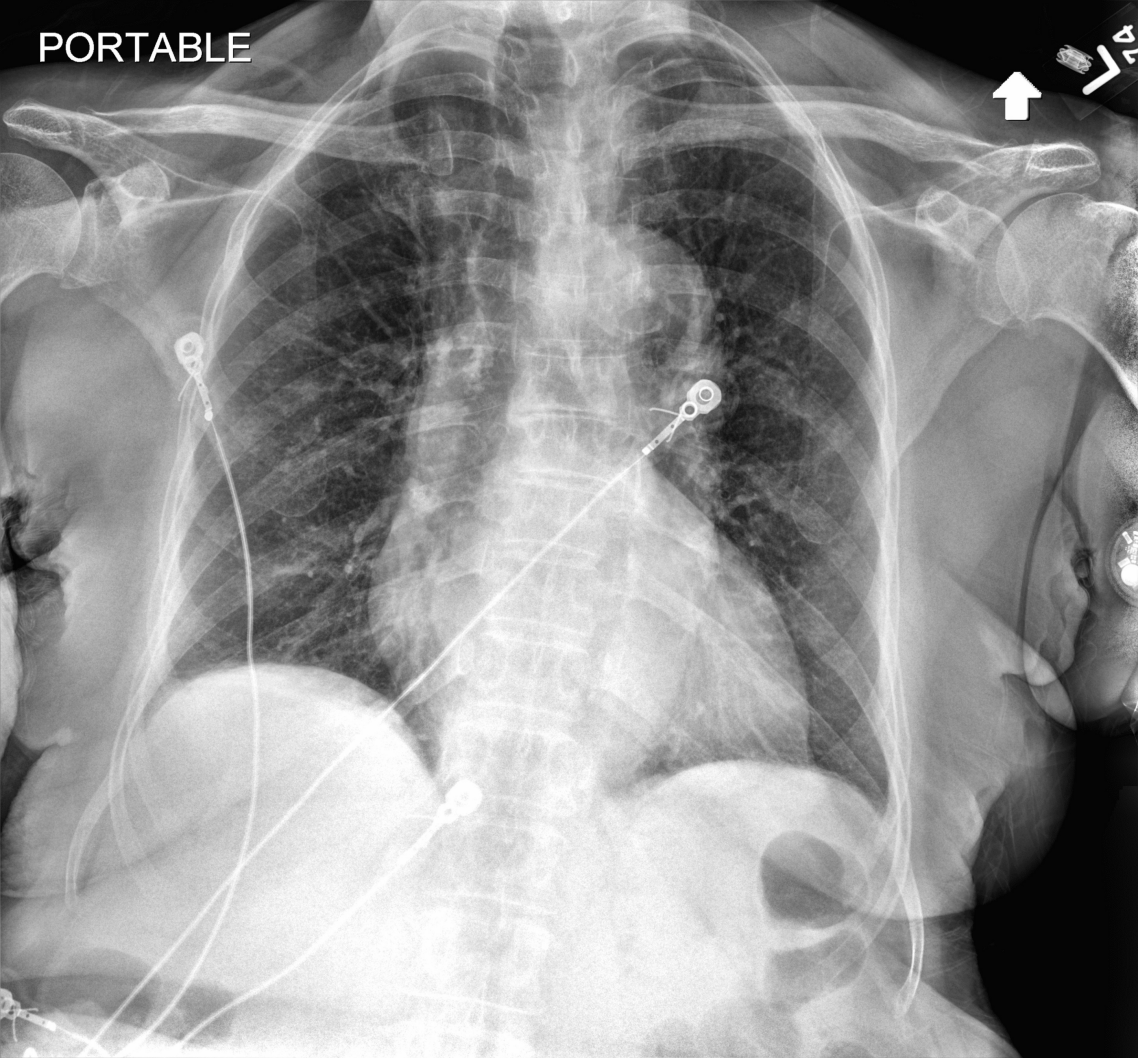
Chest X-ray on 12/08/2023 The cardiac silhouette is within normal limits for technique. The mediastinum is midline. The thoracic aorta is mildly tortuous with calcifications at its arch. The lungs are well aerated showing mild hyperinflation without consolidation. No indication of edema or airspace disease.
